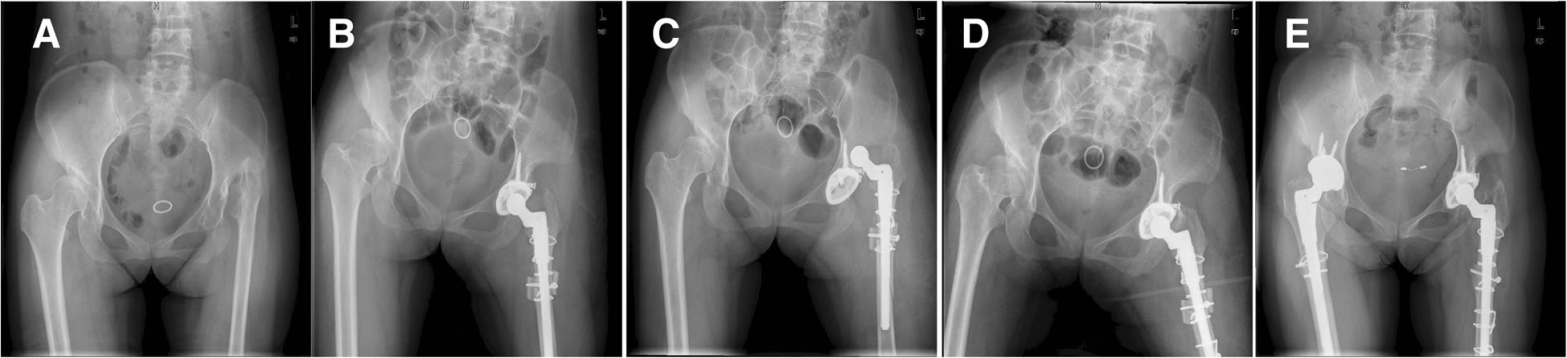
Radiographs of a 43-year-old woman with unilateral osteoarthritis secondary to hip pyogenic infection. a Preoperative anteroposterior view. b Postoperative radiographic image at 1 day. Total hip arthroplasty was performed combined with transverse osteotomy in the left hip. Fixation was conducted with wire and screw. c, d Postoperative anteroposterior view showed dislocation and distal fracture of the prosthesis at 3 days, which was treated successfully through open reduction and internal fixation. No dislocation recurred. The cause was an accidental fall. e At 9-year follow-up, the Harris Hip Score was 95. No loosening of components was found, and the femoral stem was judged to be stable with bone ingrowth. Total hip arthroplasty was performed combined with transverse osteotomy in the right hip. Fixation was conducted with wire and screw
